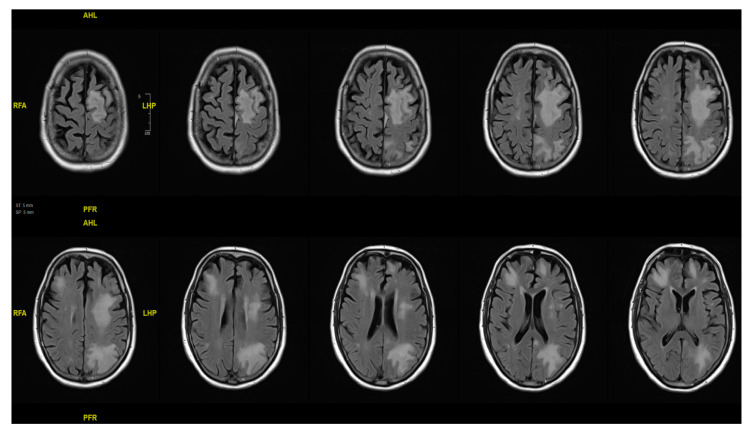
Brain MRI axial T2/FLAIR images demonstrating widespread hyperintense white matter abnormalities, predominantly in the frontal and left parietal regions, without signs of recent infarct or hemorrhage.
